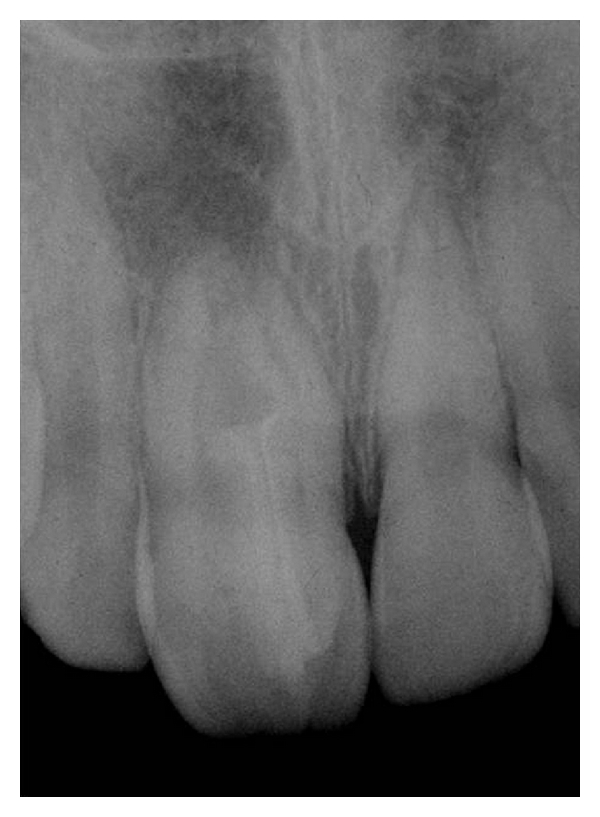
Diagnostic radiograph. Note the presence of type III dens invaginatus and a radiolucid image at the apex of tooth no. 11.
